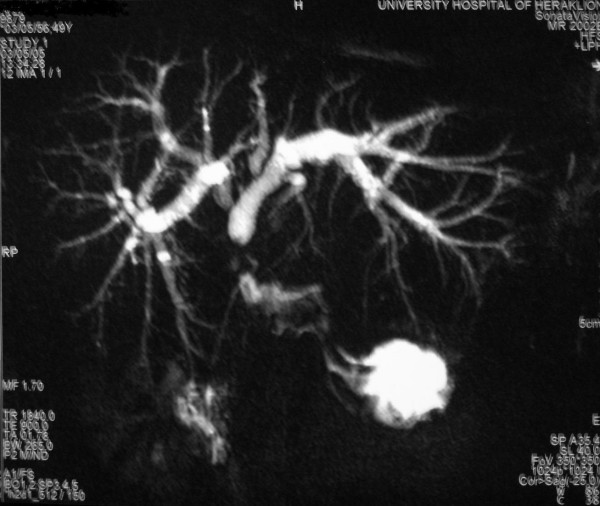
A triple bile duct injury, with right and left hepatic duct ligation and common bile duct cross-section (Bismuth type V), was revealed in magnetic resonance imaging.
